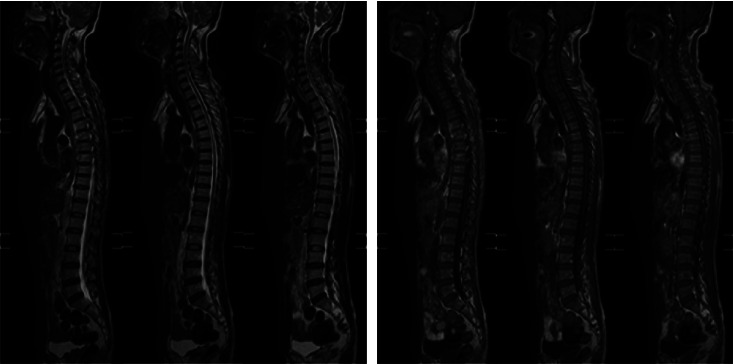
Magnetic resonance imaging of the whole spine (January 28, 2018): C4/5-C6/7 discs protrude backward, ligamentum flavum is hypertrophic, and the spinal canal is slightly narrowed; L4/5, L5/S1 discs protrude backward; degenerative changes of the entire spine.
